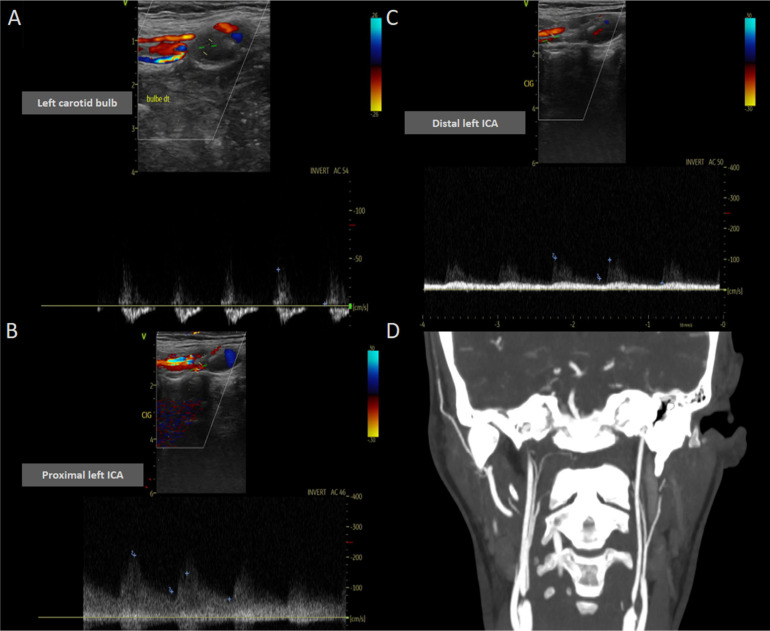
Follow-up neurosonology and cervical CTA demonstrating partial recanalization of the left ICA with persistent pre-occlusive hemodynamics. (A) Left carotid bulb Doppler shows reappearance of luminal flow but marked distal resistance, with low systolic velocity (PSV 38.1 cm/s) and near-absent diastole (EDV 2.3 cm/s; RI 0.94). (B) Proximal left ICA demonstrates high systolic velocity (PSV 190 cm/s) with preserved diastolic component (EDV 60.9 cm/s; RI 0.58), consistent with critical stenosis and turbulent reperfusion flow. (C) Distal left ICA shows persistent hemodynamic impairment with reduced systolic velocity (PSV 96.6 cm/s) and diminished diastolic flow (EDV 22.3 cm/s; RI 0.77), indicating incomplete normalization of downstream perfusion. (D) Coronal cervical CTA confirms thread-like recanalization of the left ICA rather than full-caliber restoration, supporting a pre-occlusive, critically narrowed reperfused state rather than true anatomical patency.
